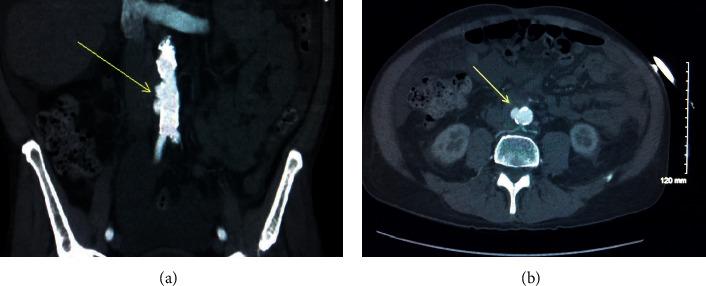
(a and b) presence of multilobulated aneurysm of infrarenal abdominal aorta with aortic wall thickening and subtle fatty stranding; aneurysm is indicated by arrows, on coronal and axial images from CT of the abdomen and pelvis with iodinated contrast.
